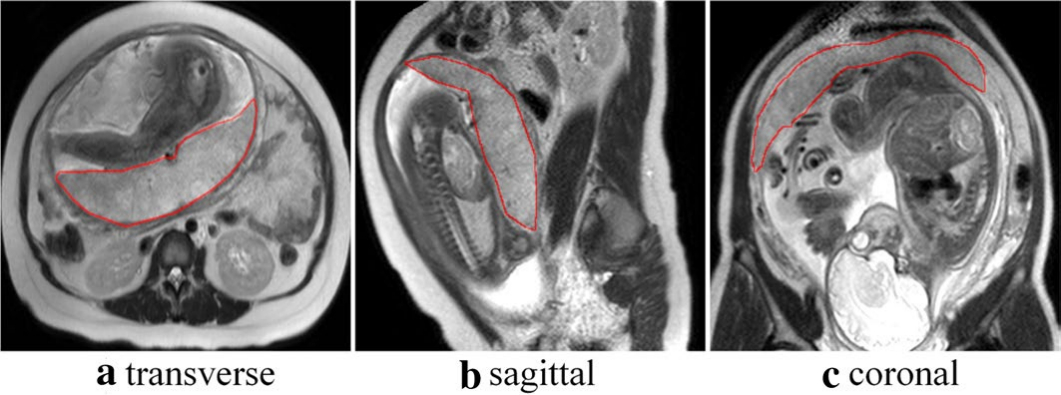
Examples of the T2WI MRI images and labels used in the present study
